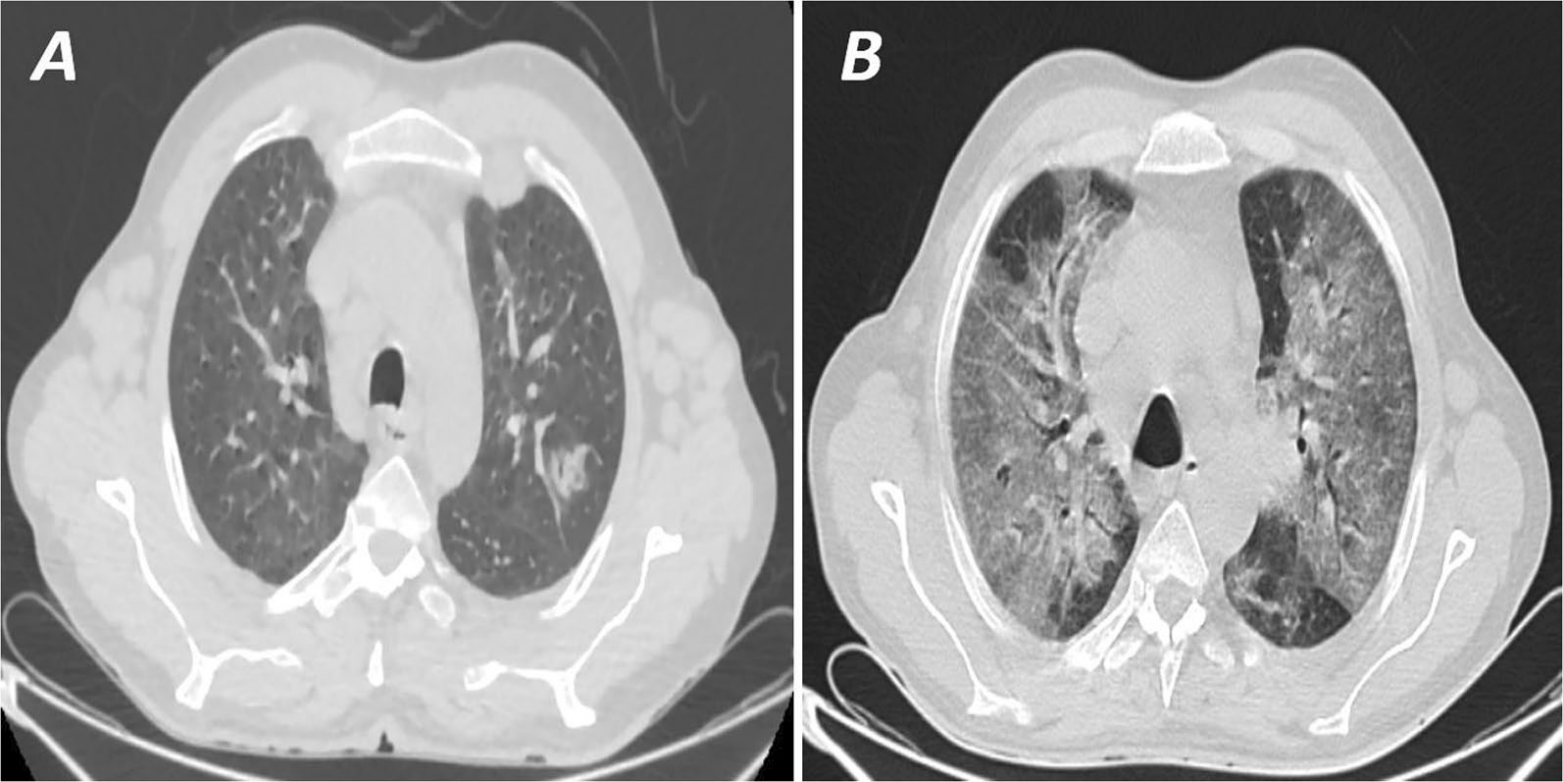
A High-resolution CT scan (axial cuts) for a 55-year-old male patient with recently diagnosed chronic myeloid leukemia presented with fever. The initial CT was interpreted as atypical for COVID (CO-RADS 2) with two patches of subsegmental consolidation in the left lobe and lingual. RT-PCR was positive for COVID-19. B CT scan was done nine days later after the patient developed desaturation shows a picture of non-cardiogenic pulmonary edema (ARDS), and the patient died a few days later
